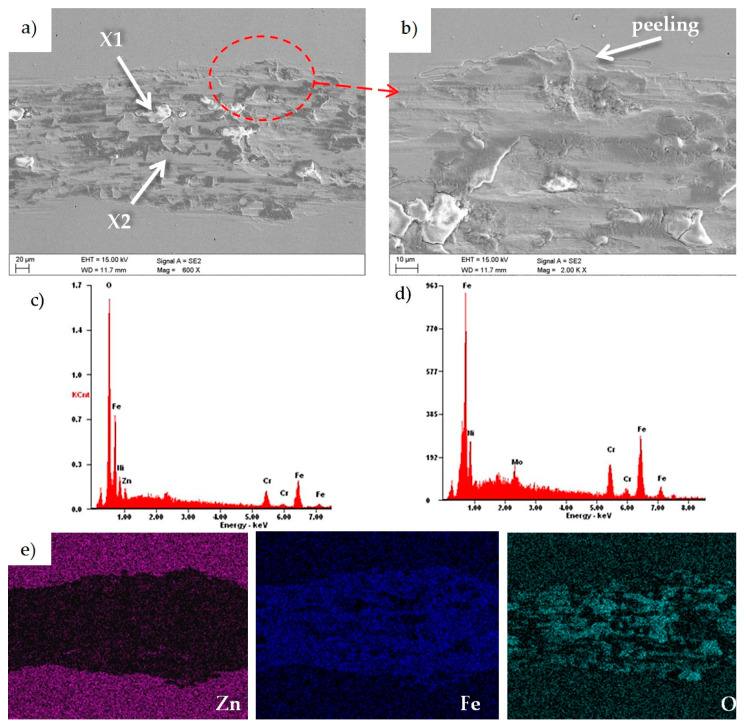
(a,b) Wear trace after the “ball-on-plate” wear test for the ZnO layer deposited in 1500 cycles, (c) X-ray energy dispersive plot of the area X1 shown in Figure 15a, (d) X-ray energy dispersive plot of the area X2 shown in figure, and (b,e) maps of superficial distribution chemical elements from the area shown in Figure 15a.
